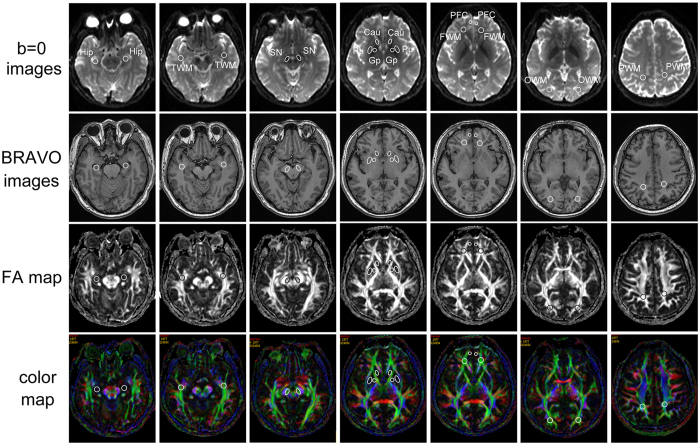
Region of interest (ROI) settings. Cau, caudate nucleus; FWM, frontal white matter; Gp, globus pallidus; Hip, hippocampus; OWM, occipital white matter; PFC, prefrontal cortex; PWM, parietal white matter; Pu, putamen; SN, substantia nigra; TWM, temporal white matter. The individual regions are drawn on DTI MR images (4,600/82.9) on the fractional anisotropy (FA) map, color map, b = 0 image and BRAVO map (8.2/3.2).
